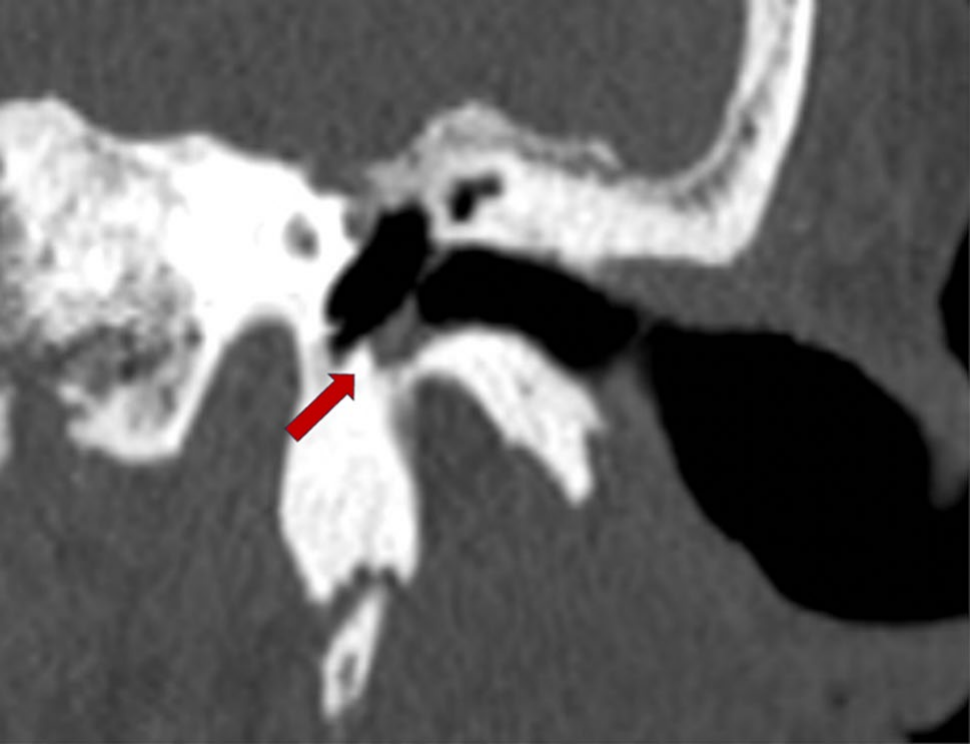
CT left temporal bone coronal plane. Excavation of EAC floor and tissue accumulation at the inferior insertion of the tympanic membrane (arrow)
